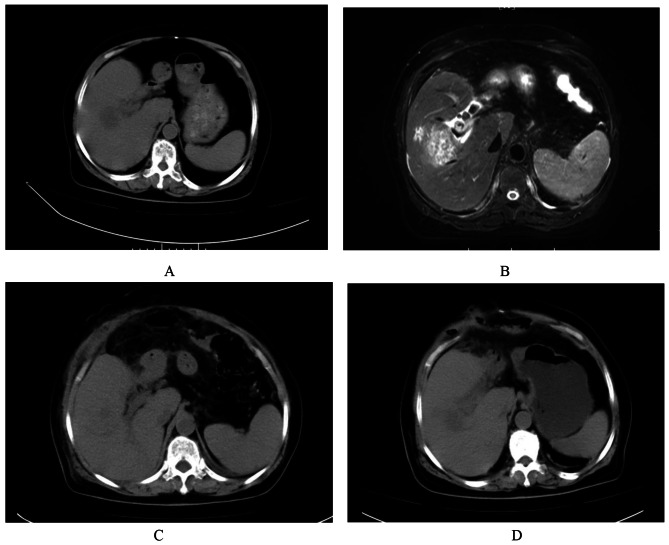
A) September 17th CT; B) NMR images showing low density lesion in the right lobe of the liver with circular enhancement, suggesting a high possibility of liver abscess and choledocholithiasis. C) October 1th CT scan indicates changes after common bile duct stone removal surgery; The scope of the abscess in the right lobe of the liver has decreased compared to before; D) October 5th CT images showing gas accumulation and exudation around the gastric antrum
